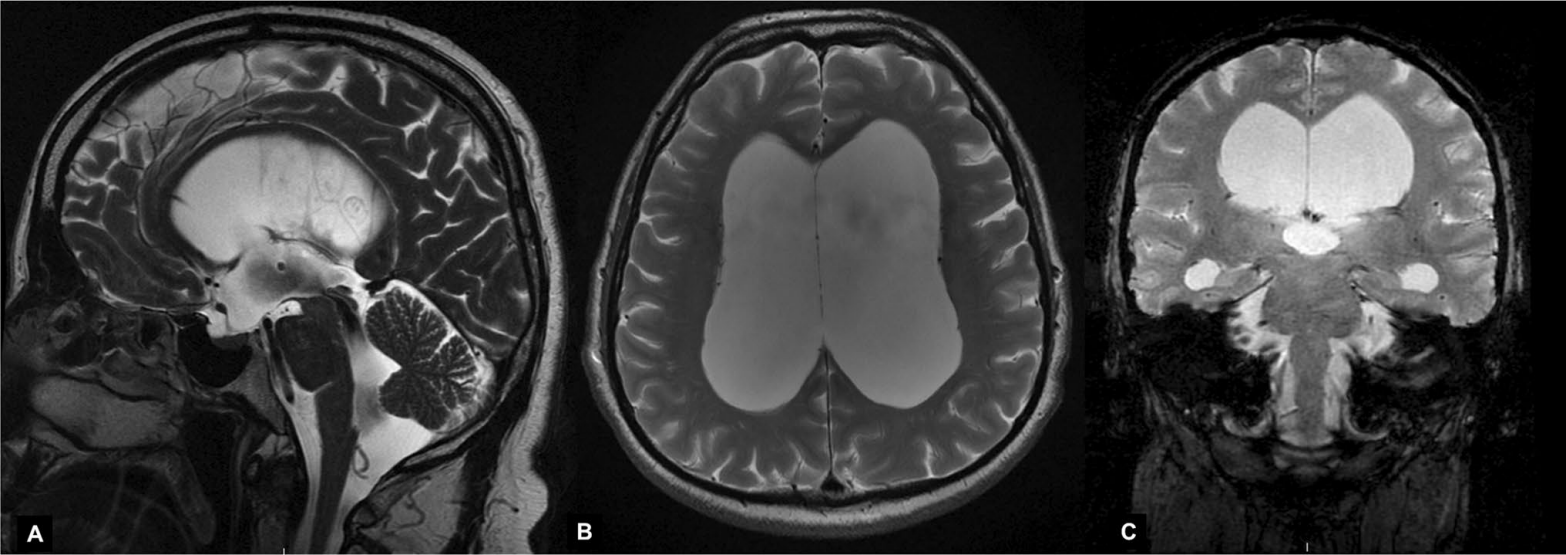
T2-weighted MRI showing peculiar features of LOVA in midsagittal (A), axial (B), and coronal view. A Distorted sellar bone, bulging of the third ventricle floor, patent aqueduct with turbulent flow, and an enlarged cisterna magna are observed. B, C A concomitant severe ventriculomegaly is reported. Evans index: 0.48. Third ventricle width: 21 mm
